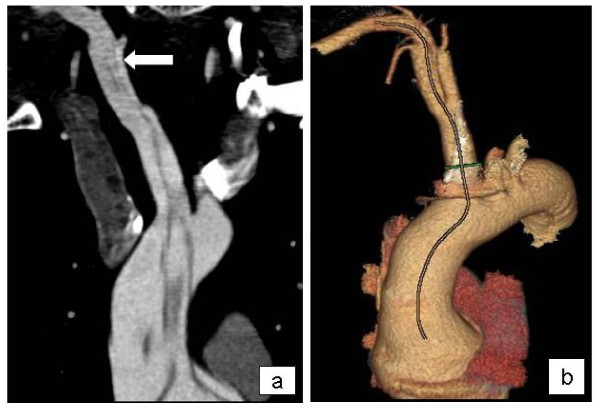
Contrast-enhanced CT scan showing the intimal flap due to dissection from the aortic root to the ascending aorta, innominate artery and subclavian artery (a). Enhanced reconstructed CT scan image showing the path of dissection (b).
